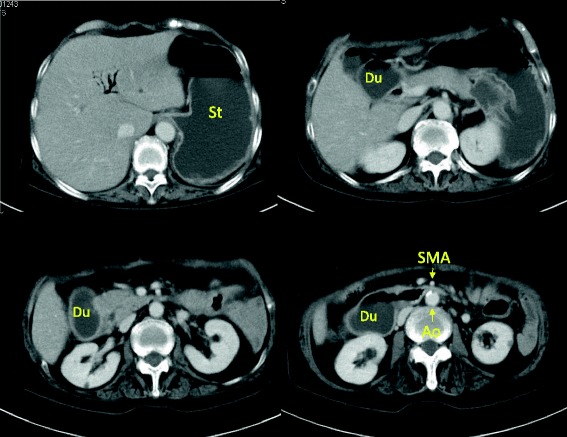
CT on postoperative day 97. CT scan demonstrated gastric and duodenal distension and a transition point in the third portion of the duodenum near the takeoff of the SMA. Du, duodenum; St, stomach; Ao, aorta; SMA, superior mesenteric artery.
